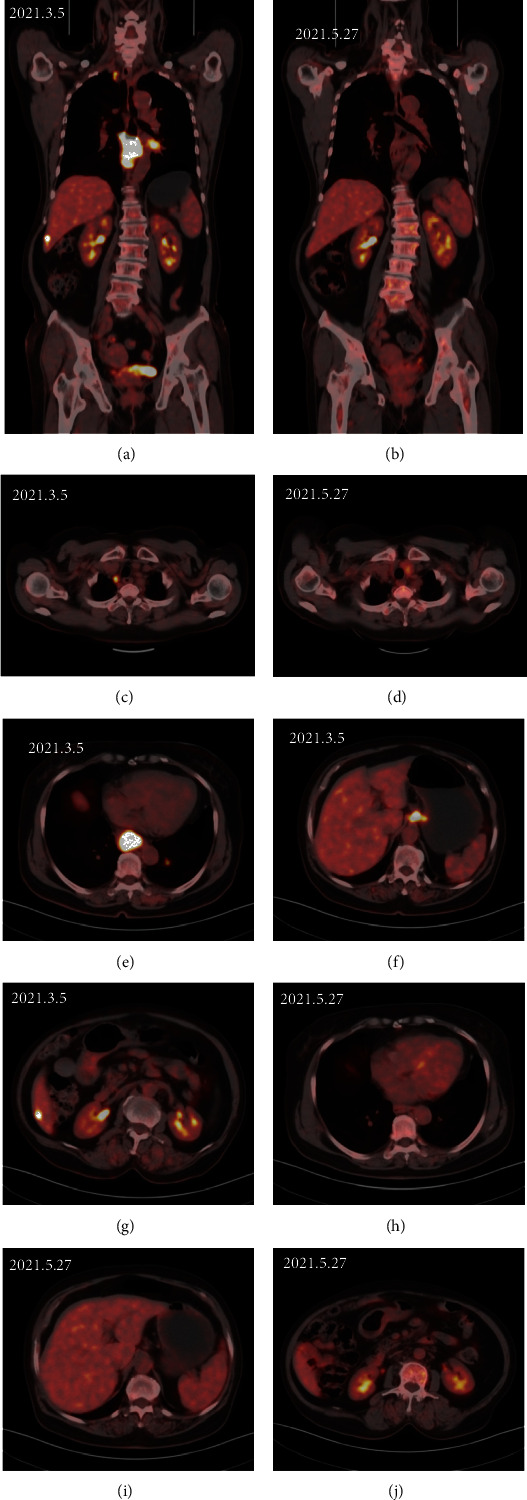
Comparison of imaging findings in a patient who was diagnosed with advanced esophageal cancer with lung and liver metastases at the first visit and received sintilimab-based regimens. (a, c, e–g) Imaging findings before treatment with 2 cycles of sintilimab plus albumin-bound paclitaxel, nedaplatin, and palliative radiotherapy for esophageal tumors (5 March 2021). (b, d, h–j) Positron emission tomography-CT showed that all lesions and tumor metabolic activity disappeared after treatment (27 May 2021).
